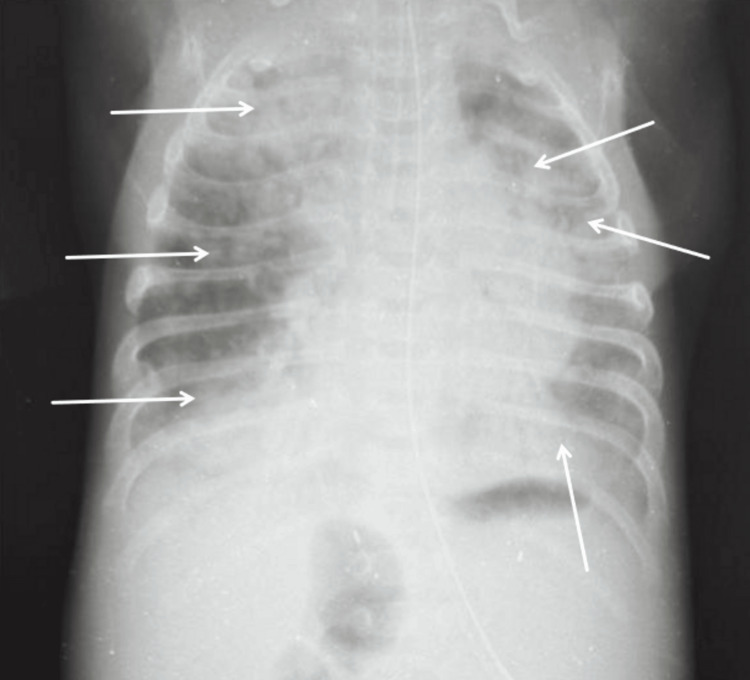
Chest radiograph (day 24) depicting marked deterioration with diffuse bilateral opacification
